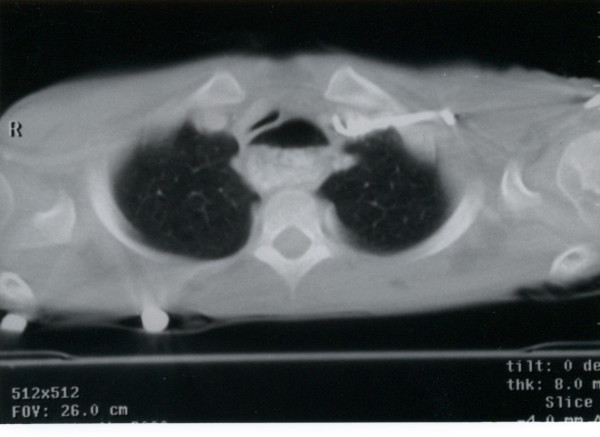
CT scan of chest showing dilatation of esophagus with air-fluid level. Dilated esophagus is compressing the trachea anteriorly.
